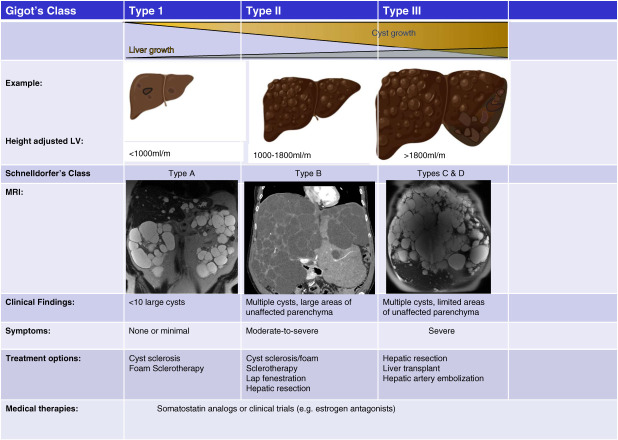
Classification criteria for PLD severity, clinical presentation, symptoms, and treatment options. Gigot and Schnelldorfer classifications are based on the number and size of the cysts and the remaining liver parenchyma. Height adjusted liver volume criteria: <1000, 1000–1800, >1800 ml/m. Coronal T2-weighted MRI images are shown for each patient type. In type A, the patient has large kidney cysts, but well-preserved liver parenchyma; in the type B patient, there is a residual preserved area of liver parenchyma; and in the type C and D patient, there is very little nonaffected liver. LV, left ventricle; MRI, magnetic resonance imaging; PLD, polycystic liver disease.
